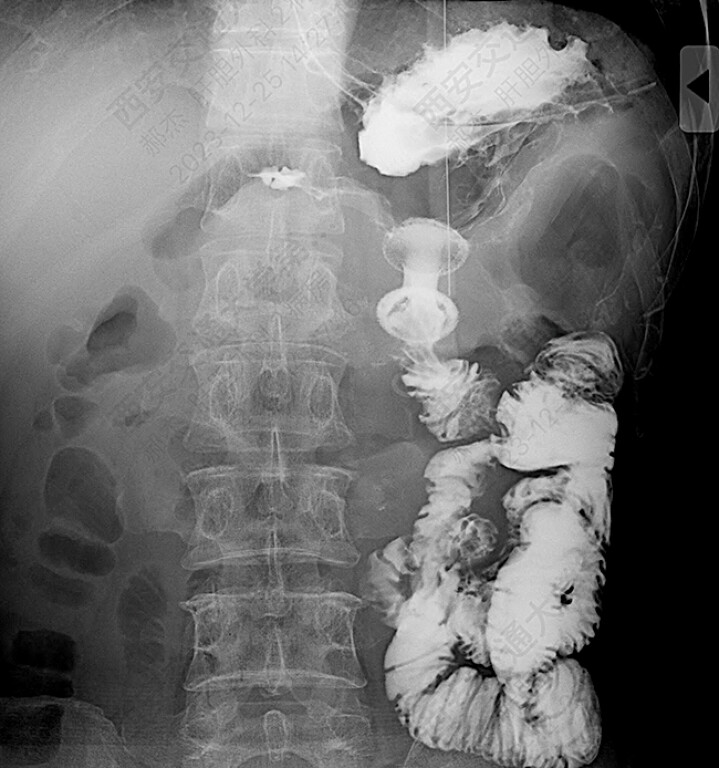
Postoperative upper gastrointestinal radiography confirmed the patency of the stent.
